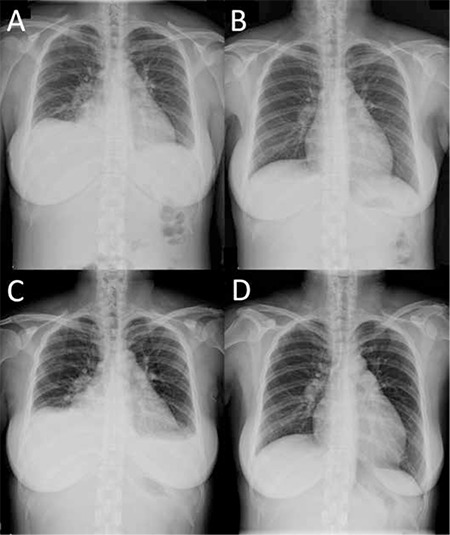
Chest radiography: A) initial small pleural effusion; B) 9 days later after diuretics and steroid treatment; C) recurrent pleural effusion after dasatinib was resumed; D) 6 weeks after dasatinib interruption.
